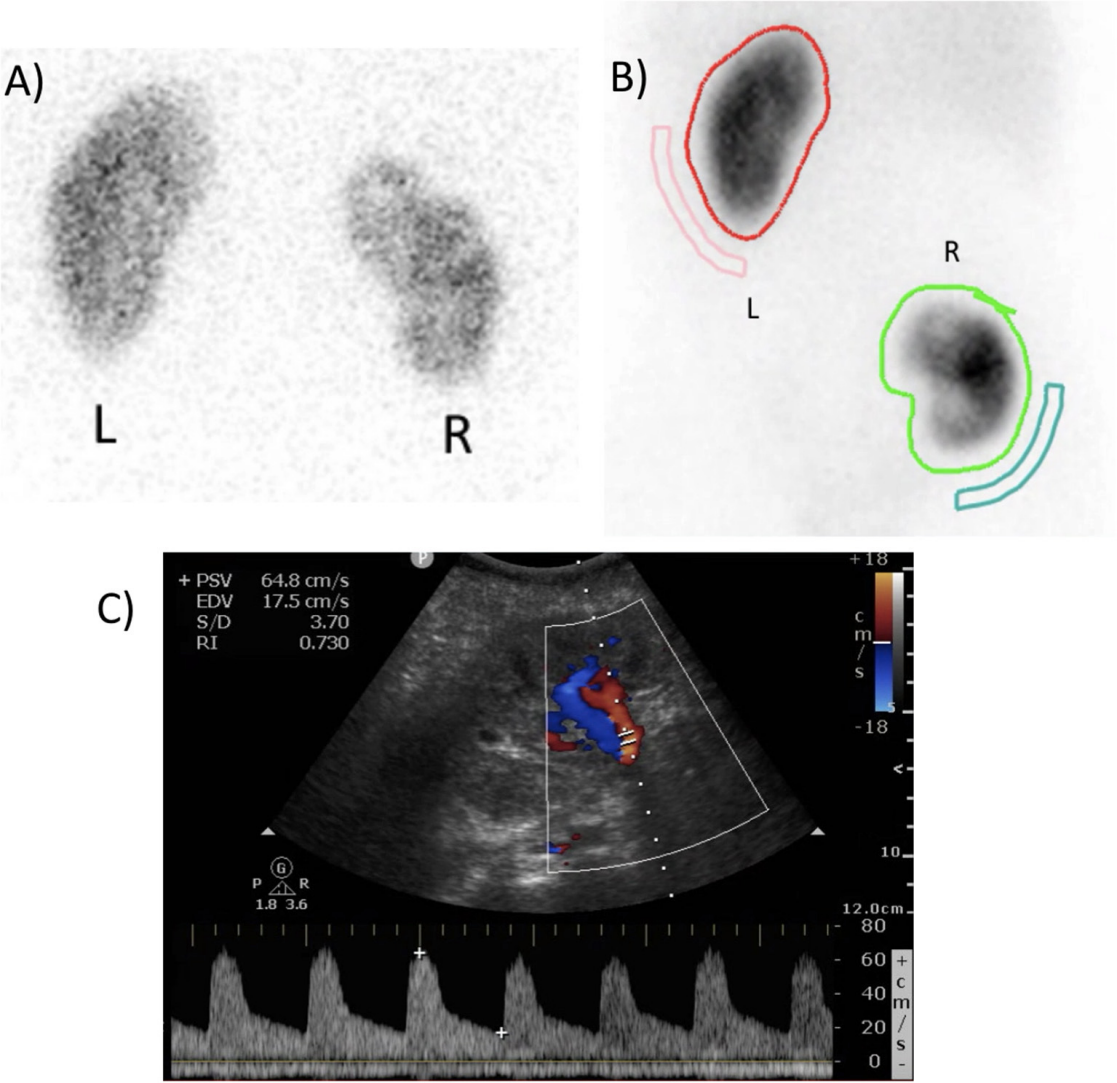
(A) Pre-operative dimercaptosuccinic acid (DMSA) renal scintigraphy showing a relative right kidney function of 43%. (B) Postoperative DMSA renal scintigraphy after 7 months, showing a relative autotransplant kidney function of 42%. (C) Postoperative duplex ultrasound of the autotransplant, demonstrating adequate perfusion with a resistance index of 0.730.
